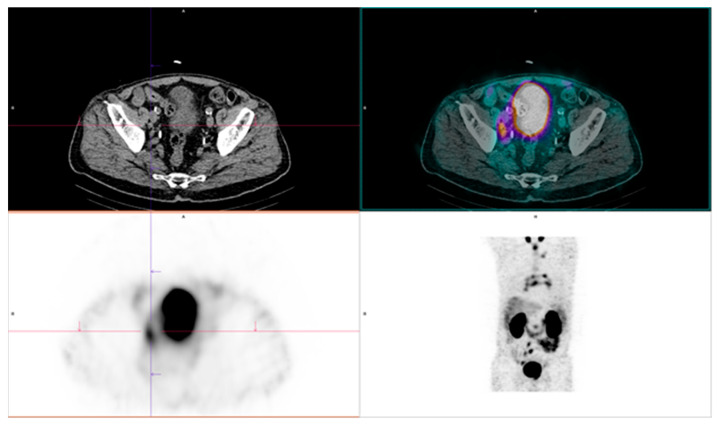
Positive findings of [^99m^Tc]Tc-PSMA. Disease is present in the right iliac lymph nodes, the retroperitoneal lymph node, and the mediastinal lymph node. Original clinical data sourced from University Clinical Center of Serbia, Center of Nuclear Medicine and PET.
